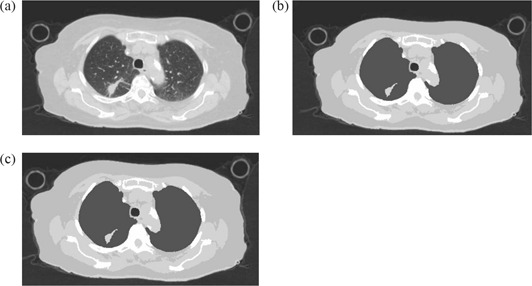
Examples of images used for creating the three plans. an original full‐resolution CT image (lung window); (b) a CT image where the electron density of each 5 bulk density was forced to be the same as each patient's average electron density of each bulk density area (IndAv.); (c) a CT image where the electron densities of each 5 bulk density was forced to be same as the average electron density of each bulk density area for the entire population (PopAv.).
